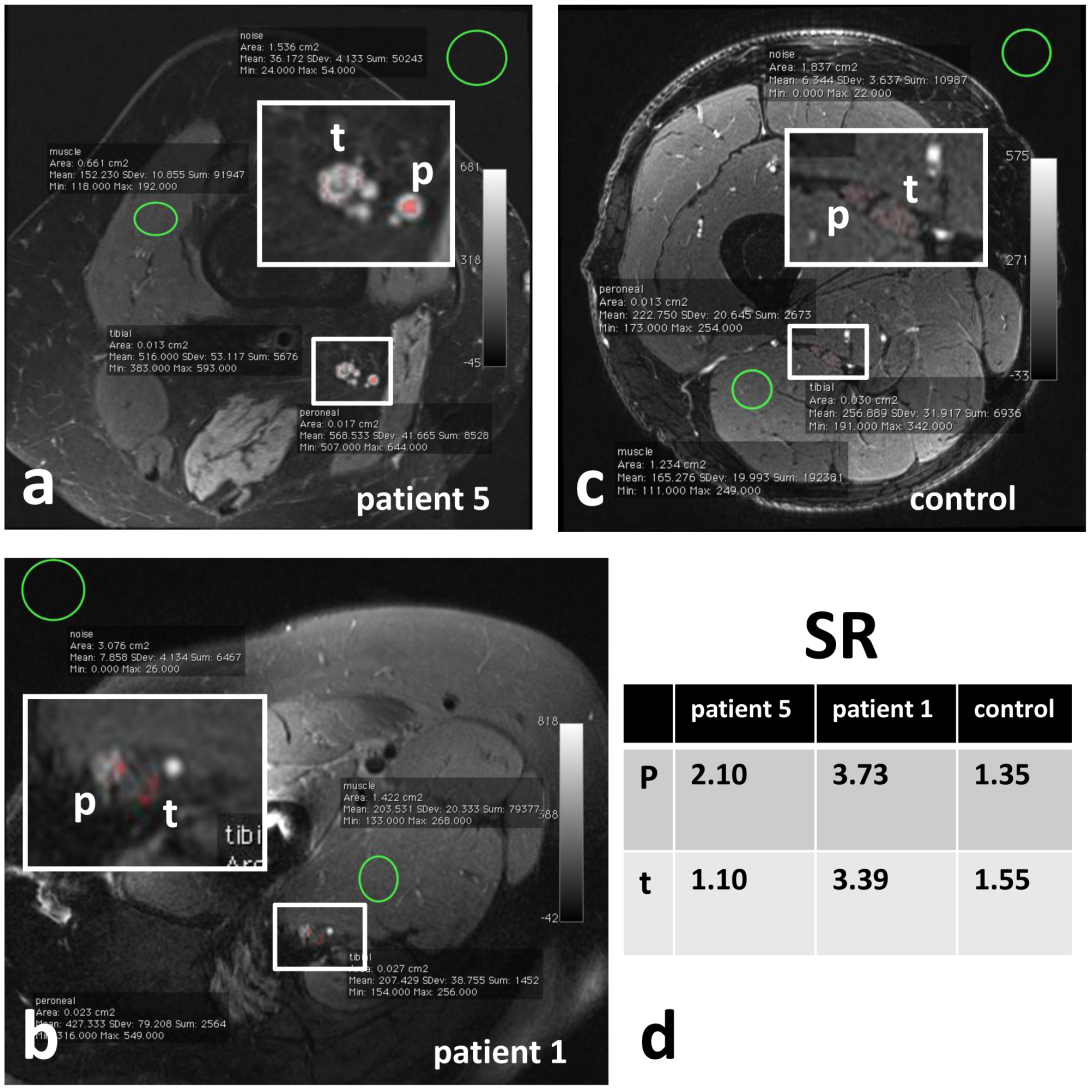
ROI-based measurement of T2-signal intensity of muscle, peroneal and tibial division of the sciatic nerve. siMR neurographic T2-weighted images with fat-saturation (a, b, c). Exemplary ROI-based analysis of signal intensities of the peroneal and tibial portion of the sciatic nerve, the normal appearing musculature, and background noise in patient 5 (a), patient 1 (b) and a healthy control (c). In (a), a distinctive T2-signal increase of the peroneal and tibial nerve in patient 5, and in (b) a T2-signal increase of peroneal division of the sciatic nerve in patient 1 are shown. In the healthy control no pathological nerve signal increase is present (c). In (d) the corresponding SR of the peroneal and tibial portion of the sciatic nerve are shown. In the healthy control, and in the normal appearing tibial portion of the sciatic nerve in patient 1 SR are below 1.7. A SR above 1.7 indicates a lesion of the peroneal portion of the sciatic nerve in patient 1, and in the peroneal and tibial portion of the sciatic nerve in patient 2 (d). Abbreviations: p: peroneal portion of the sciatic nerve or peroneal nerve; SR: nerve-to-muscle T2- signal intensity ratio; t: tibial portion of the sciatic nerve or tibial nerve.
